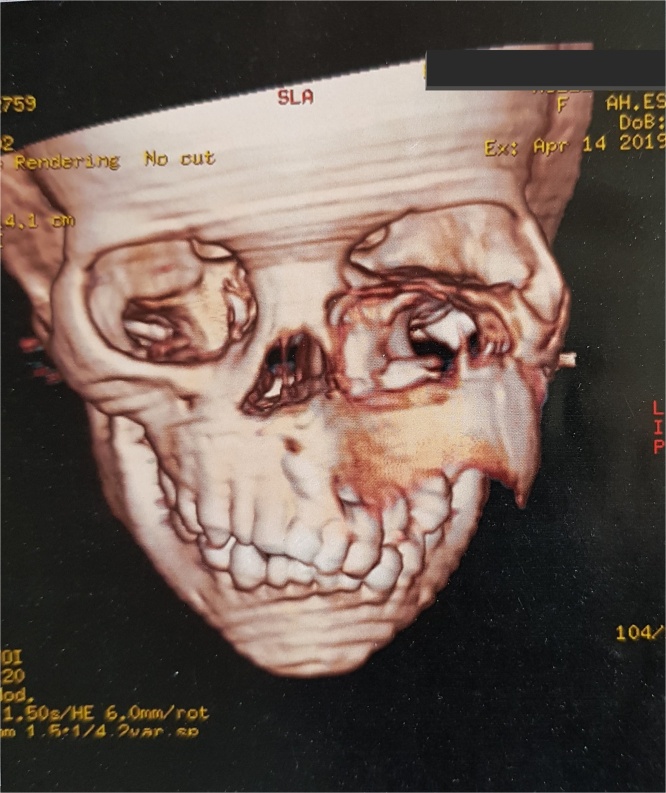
3D volume reconstructed CT showing expansile large lesion in maxilla and left orbit.
